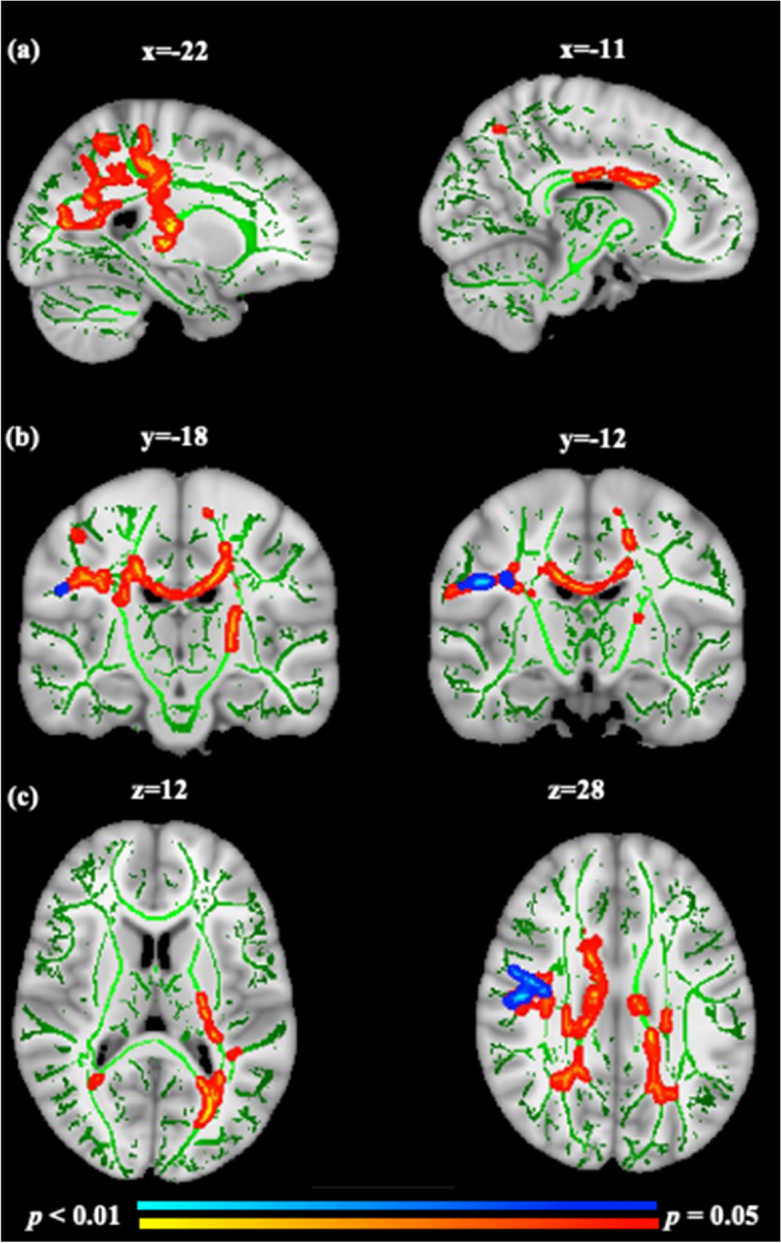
Birthweight z-scores are negatively associated with FA (red) and positively associated with RD (blue) clusters in the white matter skeleton at 9 to 10 years of age. Significant clusters are overlaid on the Montreal Neurological Institute (MNI) T1-weighted template at 1-mm thickness and on the white matter skeleton (green). Red to yellow or blue to light blue indicates the size of the *p* values after correction for multiple comparisons using Threshold-Free Cluster Enhancement (TFCE). Images are sagittal (a), coronal (b), and axial (c) planes and displayed in radiological convention in which the right side of the brain is left on the image. For visualisation purposes, clusters in the whole brain skeleton with significant differences were thickened using tbss_fill towards the full width of the white matter tracts.
